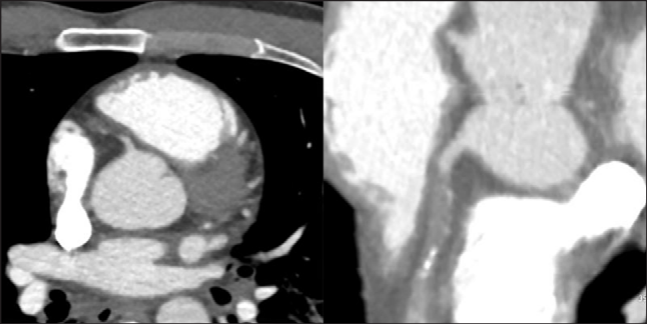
Spiral acquisition trauma CT of the thorax and abdomen after intravenous injection of 100 ml iodized contrast. Focus on the heart showing dissection and complete occlusion of the RCA. Left image: axial view, right image: curved view.
